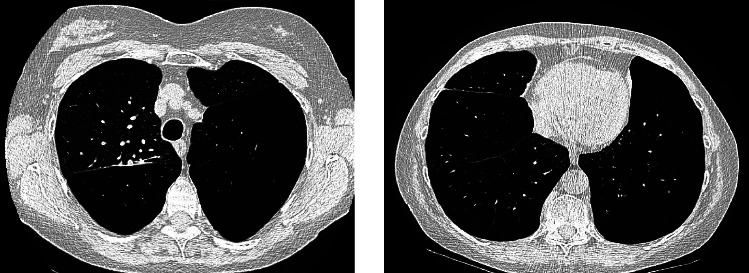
CT chest of upper and lower lobes showing panlobular emphysema with predominantly lower lobe involvement.
